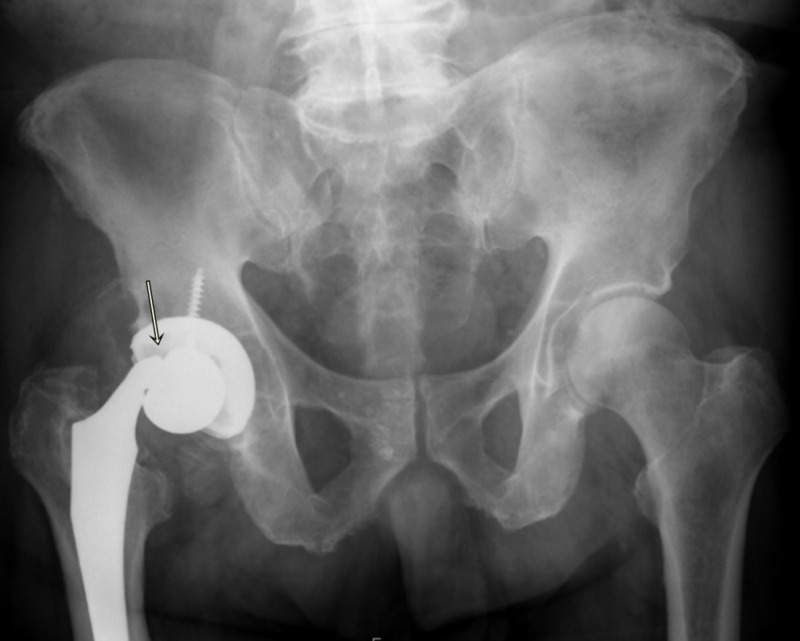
Anteroposterior radiograph of the pelvis with head neck dissociation of the right hip implant
